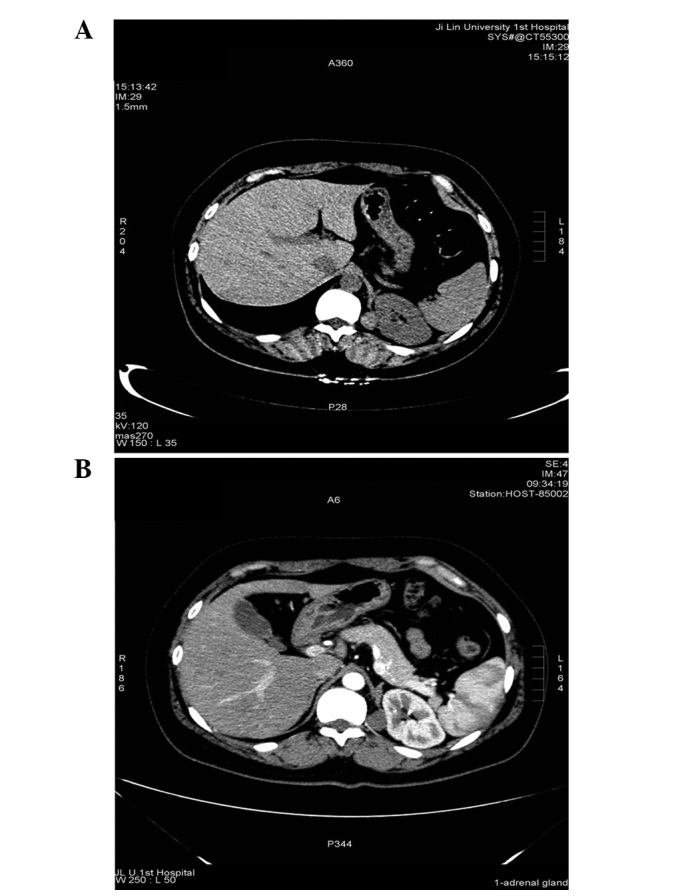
(A) Computed tomography (CT) scan revealing a cystic mass in the retroperitoneal area located medially to the left adrenal gland. (B) Contrast enhanced CT scan revealing a homogeneously dense cystic mass in the left suprarenal region.
